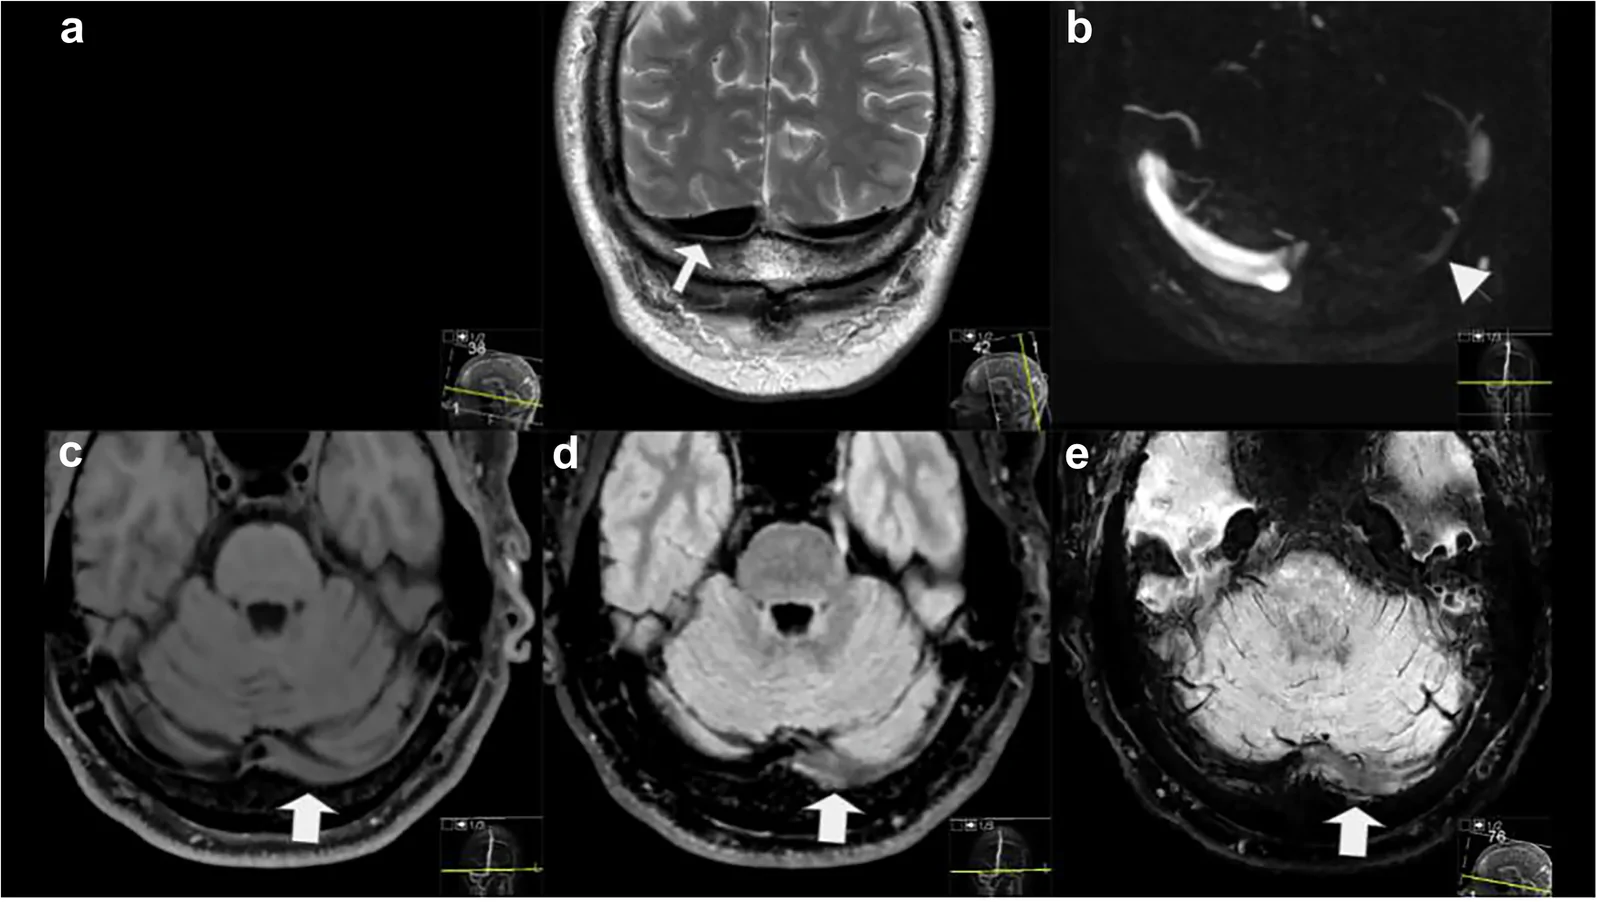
Slow flow mimicking thrombosis. Upper row: (a) T2 coronal with dark signal, due to loss of signal in high flow regions, most prominent in the right transversal sinus, and (b) phase contrast MRV shows apparent signal loss in the left transverse sinus (arrowhead), initially raising suspicion for thrombosis. Lower row: (c) T1 CUBE with fat saturation, (d) T2-FLAIR, and (e) SWI images with slight signal changes in the left transversal sinus (fat arrows)
